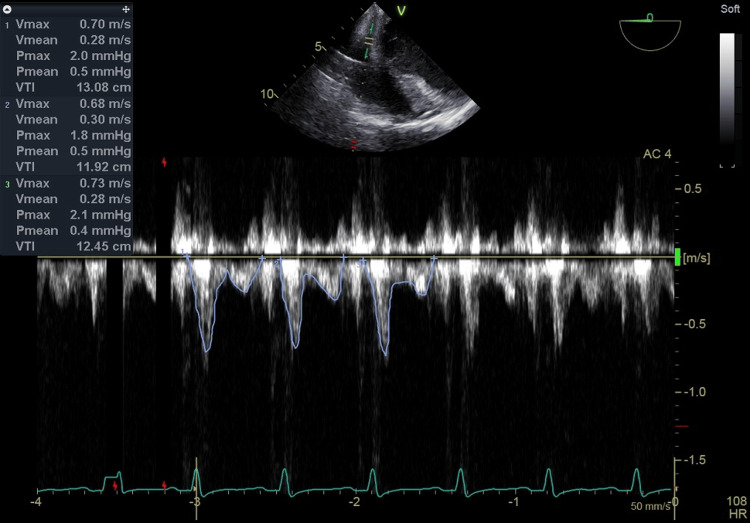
The VTI of CS blood flow measured by the TEE pulse Doppler before chest closure The VTI value before chest closure was 12.48 cm (HR 108 beats/min) calculated as the average of three heartbeats excluding premature contractions. In addition, the TEE setting was set to Frames per second 9, Frequency 3.1 MHz, Power 0 dB, Gain -2 dB, Velocity scale 2.5 m/s, Sample volume 4.0 mm, Sample volume depth 2.8 cm and Sweep speed 50 mm/s. VTI: velocity time integral, CS: coronary sinus, TEE: transesophageal echocardiography
